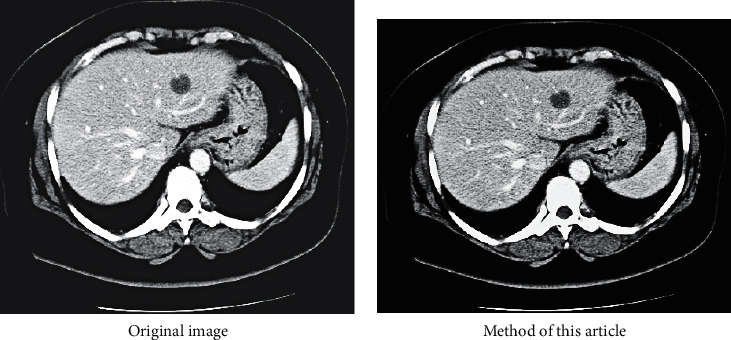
The comparison results of liver CT(Some of the pictures are from Baidu).
